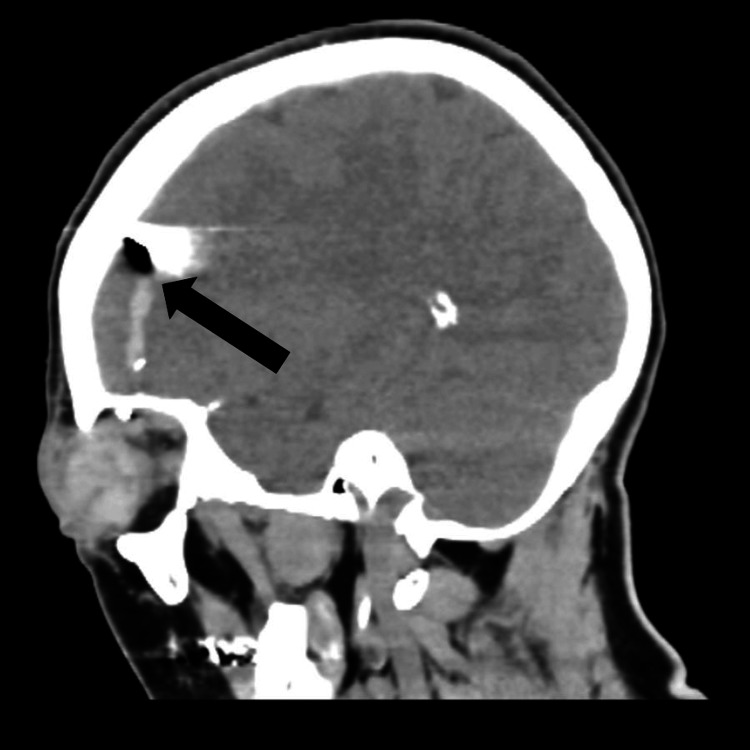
Intracranial foreign body and associated hematoma following air bag deployment: sagittal CT imaging CT: computed tomography Sagittal CT images reveal a small intracranial hematoma, located just caudal to a more rostrally positioned foreign body. The injury occurred following air bag deployment, with the foreign object penetrating the cranial cavity and resulting in localized hemorrhage
